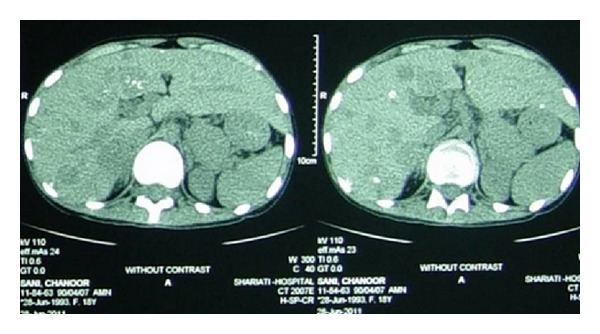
Spiral abdominopelvic CT scan (with contrast). The report was as follows. Multiple calcified and noncalcified lesions in liver are seen (metastasis should be considered). Some of the small bowel loops have thickened wall. Mild right side hydronephrosis is present. Anterior abdominal wall fistula is depicted. A few small paraaortic lymphnodes are seen. Mild left side pleural effusion and massive ascites were also noted.
